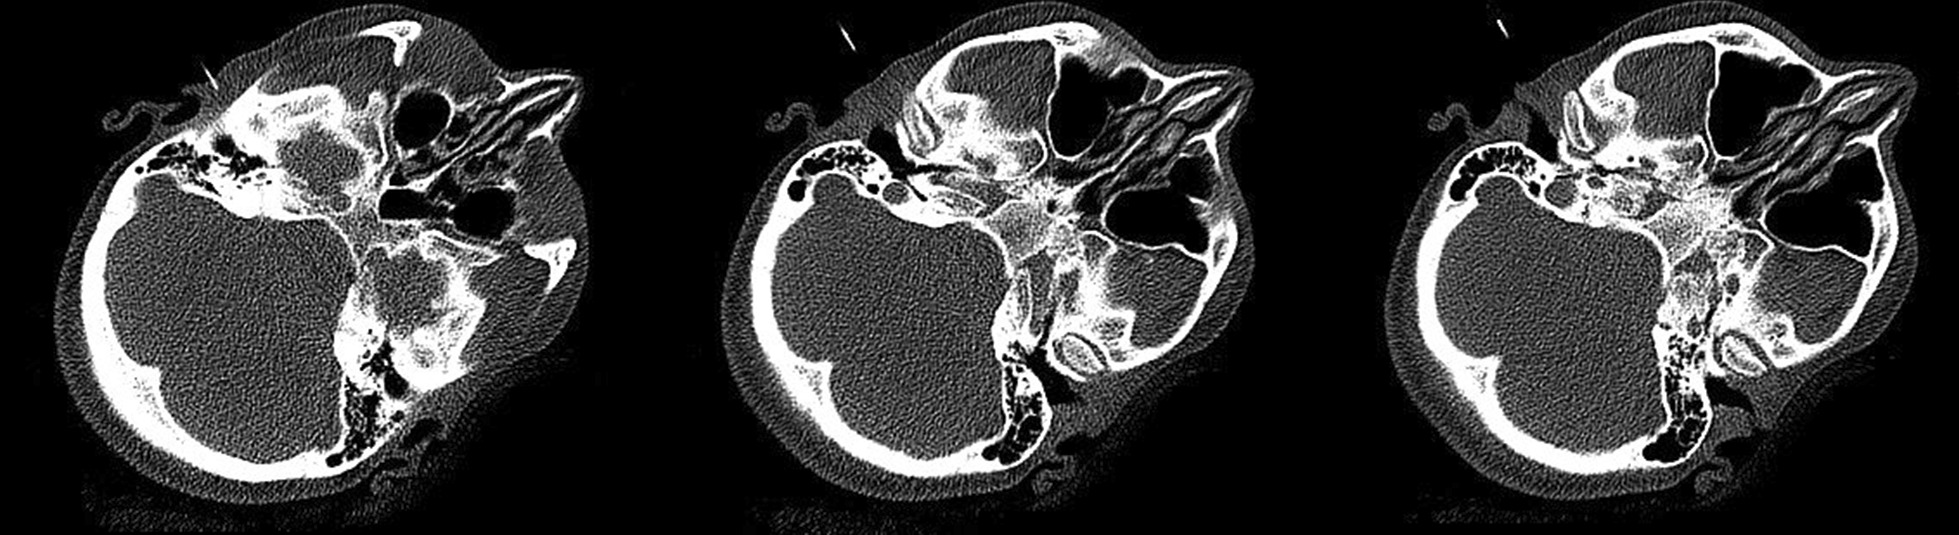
CT scan with cortisone infiltration (01/20)
